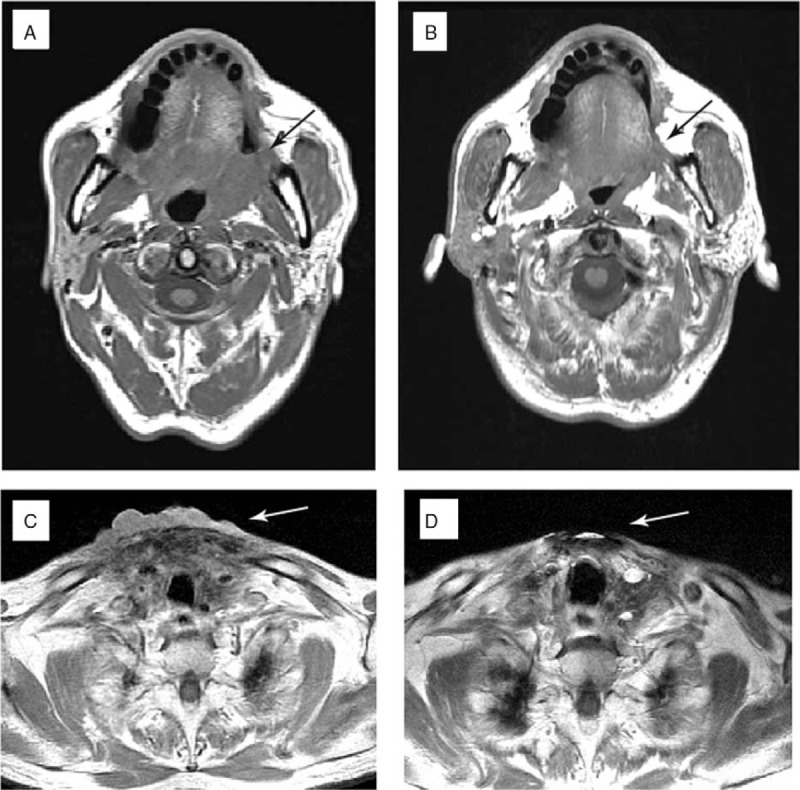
Patient No. 10 had a squamous cell carcinoma in the left hard palate, with tumor recurrence over the left palatine tonsil and invasion into the masseter muscle (A, black arrow). Complete response occurred after 6 months of treatment with pembrolizumab (B, black arrow). Patient No. 12 had a papillary thyroid cancer with surrounding invasion, and received palliative resection in 2014. Tumor recurrence (C, white arrow) in 2015 and a biopsy revealed squamous cell carcinoma, probably originating from the thyroid. A neck magnetic resonance imaging (D) showed complete response after 3 months of treatment with pembrolizumab.
